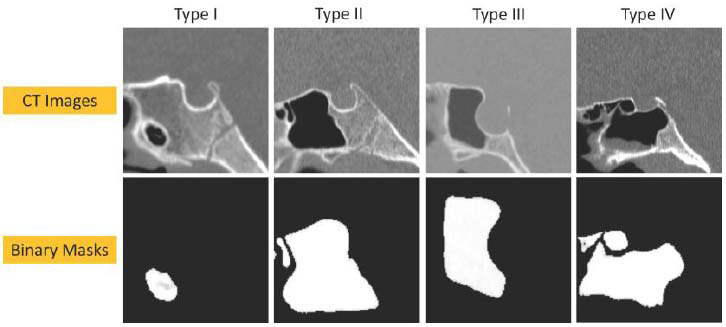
CT scan images (upper row) and corresponding binary masks (lower row) for the four pneumatization patterns of the sphenoid sinus.
